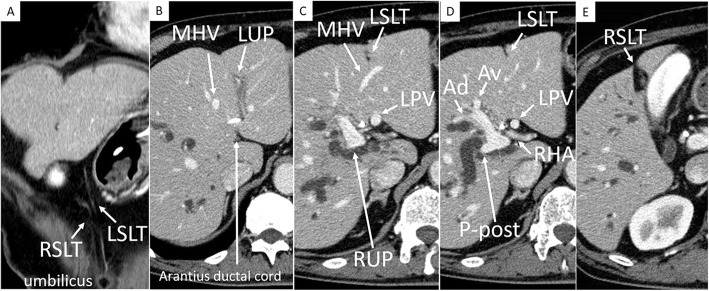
a Contrast-enhanced computed tomography (CT) image reveals that the patient had right-sided ligamentum teres (RSLT) and left-sided ligamentum teres (LSLT). b The umbilical portion of the left portal vein (LUP), to which LSLT connected, became atrophic in this patient. c The RSLT was attached to the tip of the right anterior pedicle and formed an umbilical portion of the right portal vein (RUP). d The right portal vein first ramified the posterior branch and formed the RUP, which ramified the anterior branch feeding the ventral side and the anterior branch feeding the dorsal side separately. e The RSLT was detected on the right side of the gallbladder. RSLT, right-sided ligamentum teres; LSLT, left-sided ligamentum teres; MHV, middle hepatic vein; LUP, the umbilical portion of the left portal vein; RUP, the umbilical portion of the right portal vein; LPV, left portal vein; RHA, right hepatic artery; Ad, the anterior portal vein branch feeding the dorsal side; Av, the anterior portal vein branch feeding the ventral side
